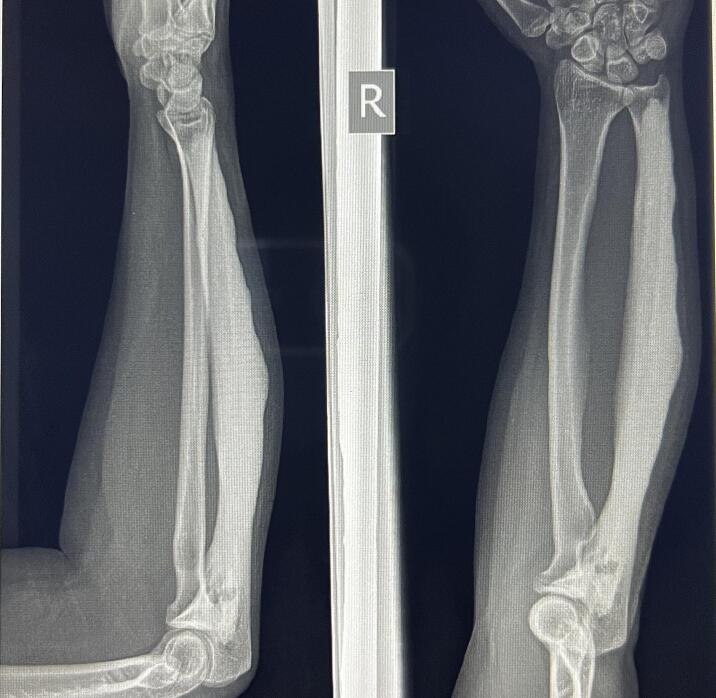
Radiograph of right forearm anteroposterior and lateral views showing cortical thickening and hyperosteotic lesion of right ulna with ‘dripping candle wax’ appearance and radial bowing of ulna.
